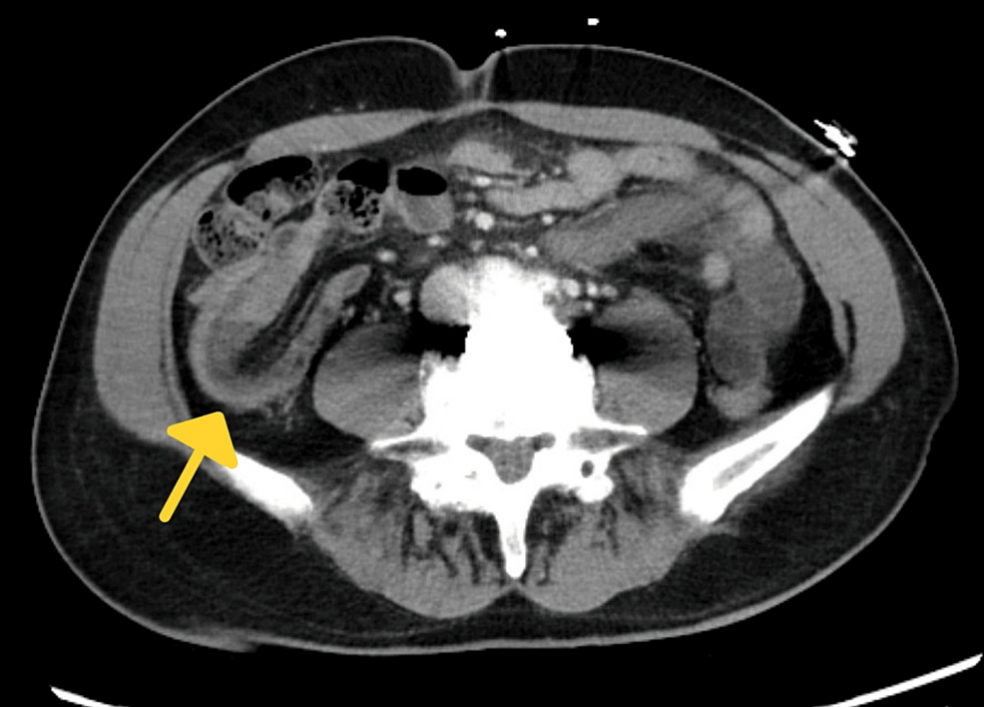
CT showing crescent sign with signs of small bowel obstruction
